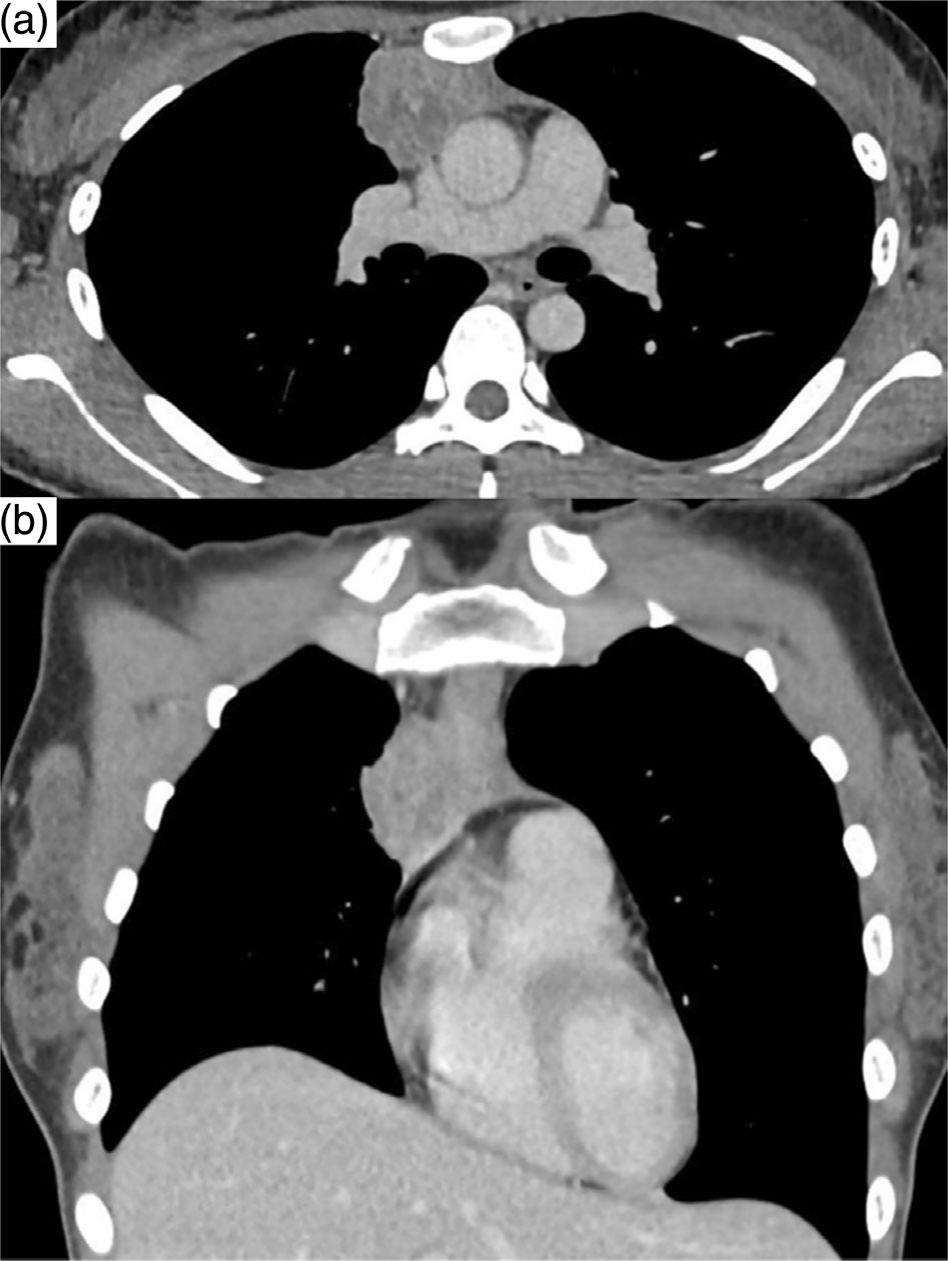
Contrast‐enhanced computed tomography of the thorax on initial examination. (a) Cross‐sectional and (b) coronal thoracic images.
